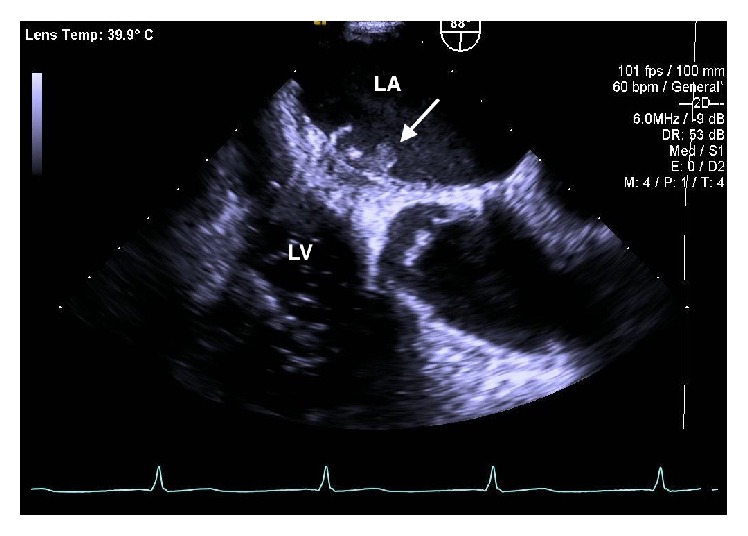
Transesophageal echocardiogram showing prosthetic mitral valve with vegetation (arrow). LA: left atrium; LV: left ventricle.
